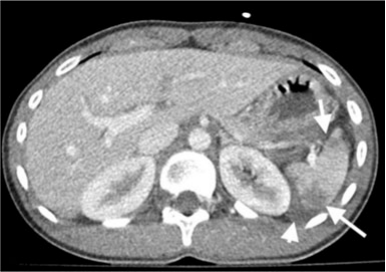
Splenic hematoma. Axial contrast-enhanced CT of a 35-year-old man after a motor vehicle accident (MVA) demonstrates hematomas in splenic parenchyma with low-attenuated appearance (*arrows*). Perisplenic haemorrhage is also noted (*arrowhead*). The splenic capsule is intact
